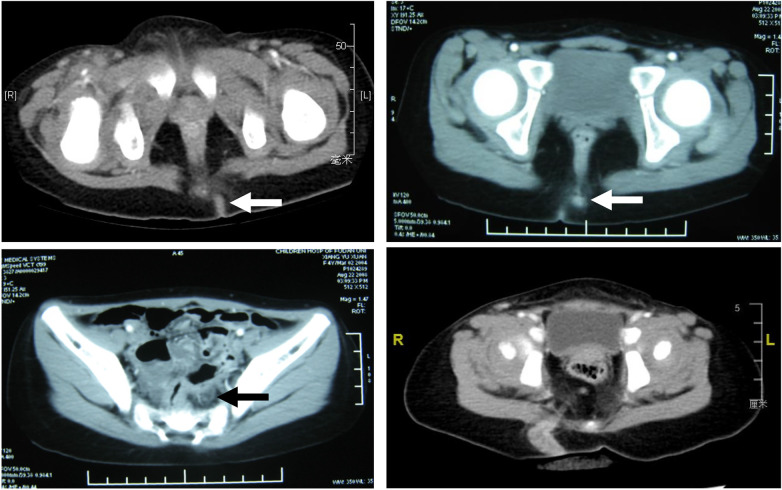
CT imaging of congenital gluteal dermal sinus tract: axial CT images of children with congenital gluteal dermal sinus tract, with arrows indicating the position of the fistula in different sequences.
